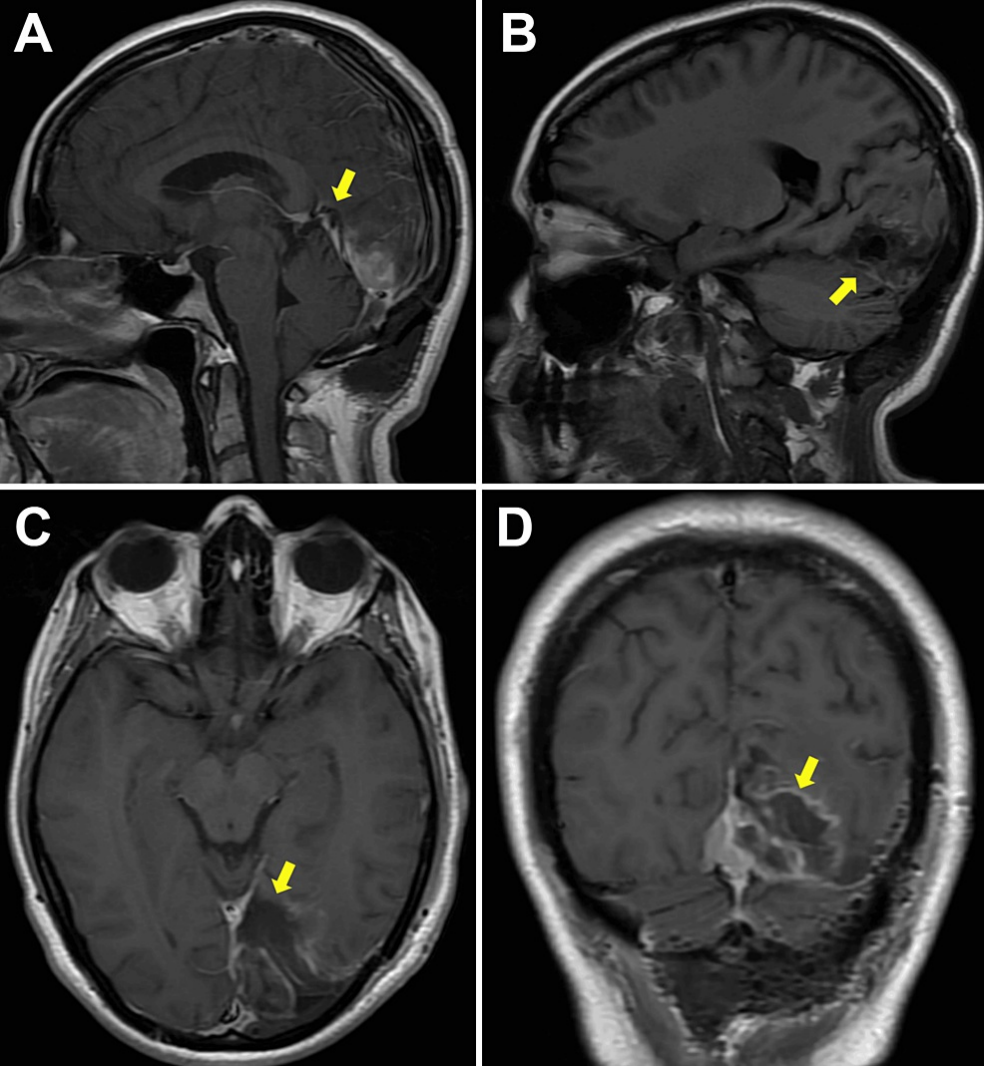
Post-surgical MRI (Panels A, B) Sagittal MRI views. (C) Axial and (D) coronal MRI views. Resection of the lesion can be observed; a residual slab remains in the medial region due to its relationship and involvement with the torcula.
